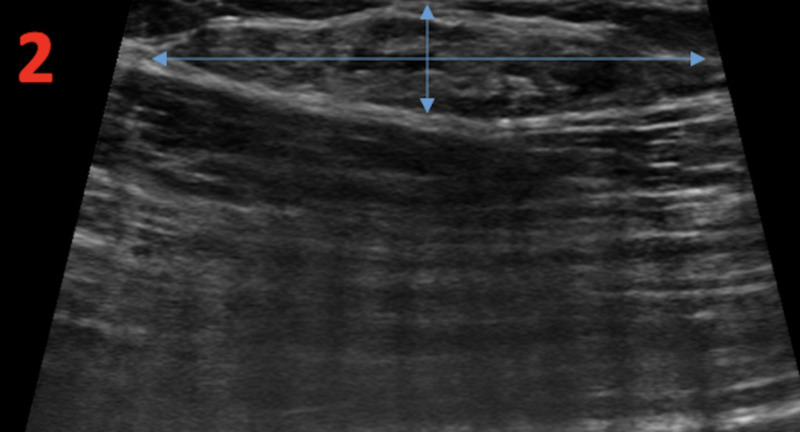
Left Breast Diagnostic Ultrasound Diagnostic ultrasound of the left breast demonstrates an isoechoic oval mass measuring 34 x 7 x 34 mm in the upper outer quadrant at a distance of 10 cm from the nipple (perpendicular blue arrows). The mass has circumscribed margins and internal vascularity.
